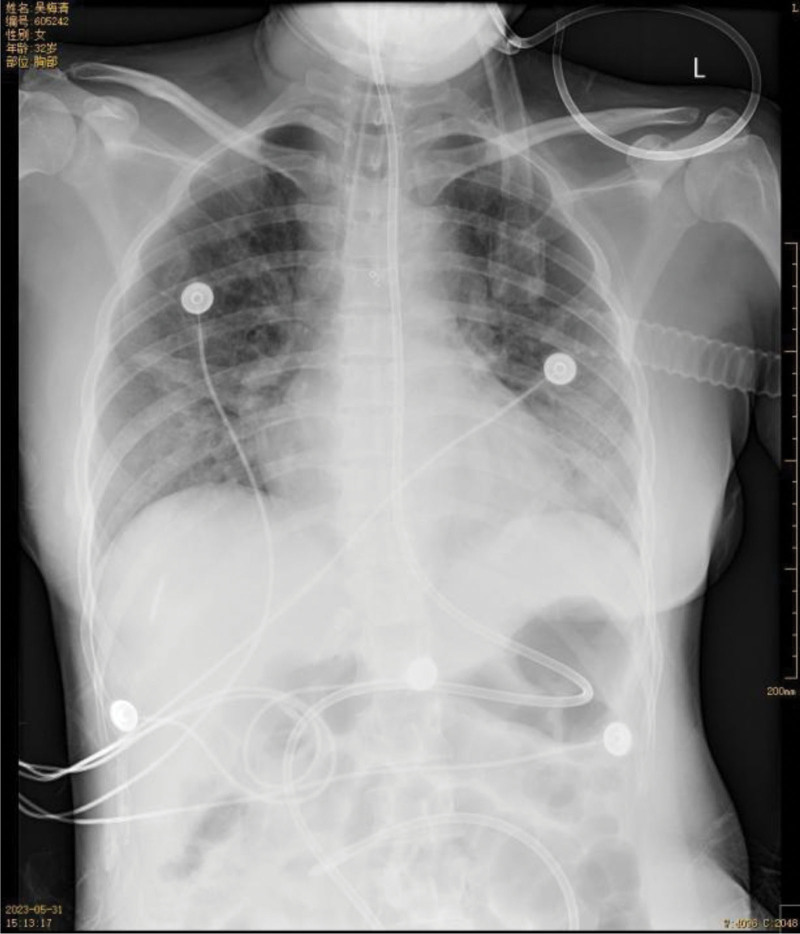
On May 31st, follow-up chest X-ray showed further improvement in bilateral obstructive atelectasis. The bilateral pneumonia and pulmonary hemorrhage had absorbed and reduced compared to previous images.
